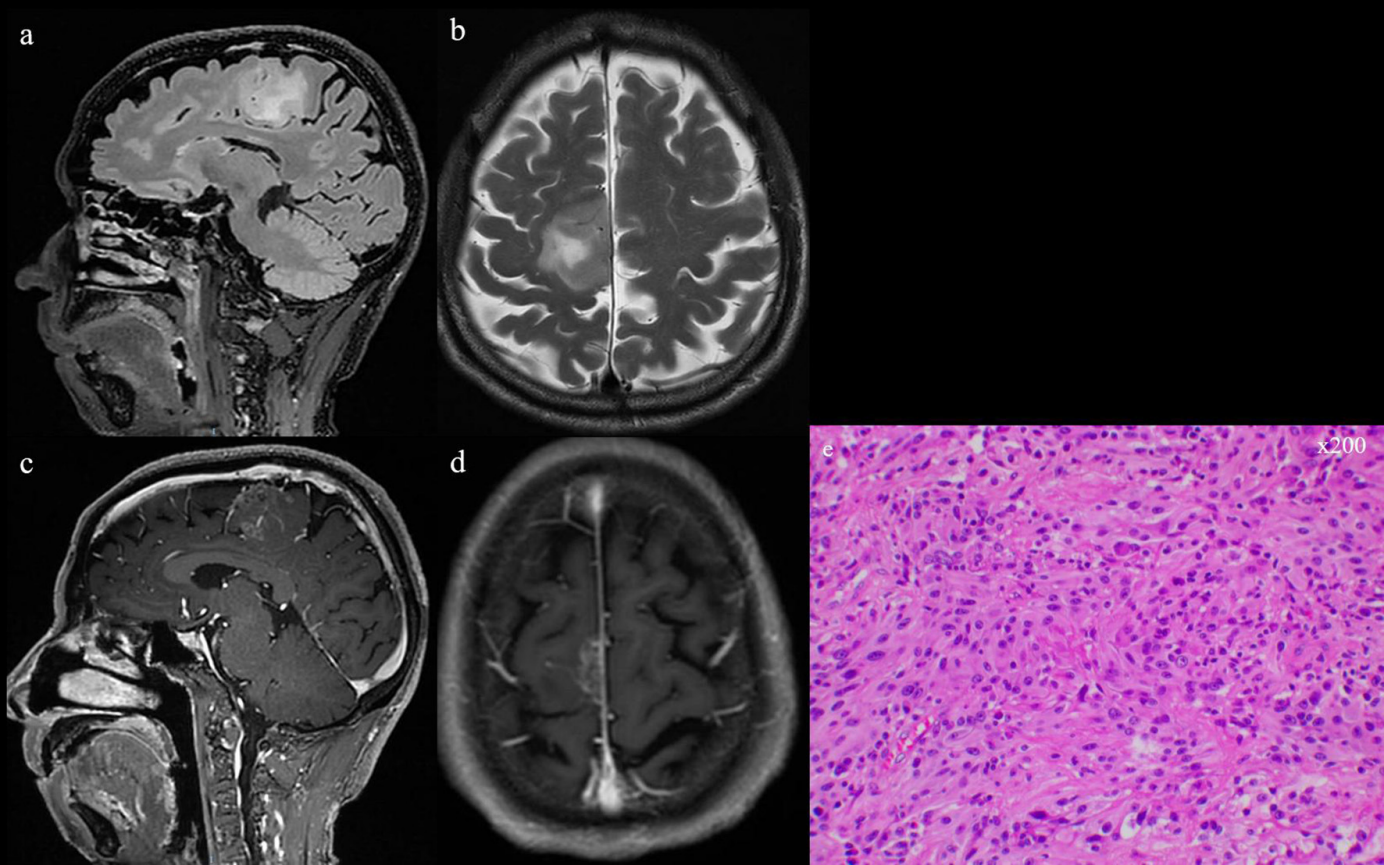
(a, b) T2 and flair series of preoperative MRI scans. (c, d) Preoperative MRI scan with gadolinium-based contrast agent, showing the occupying lesion in the left frontal lobe. (e) GBM confirmed by intraoperative pathologic examination (He, ×200).
